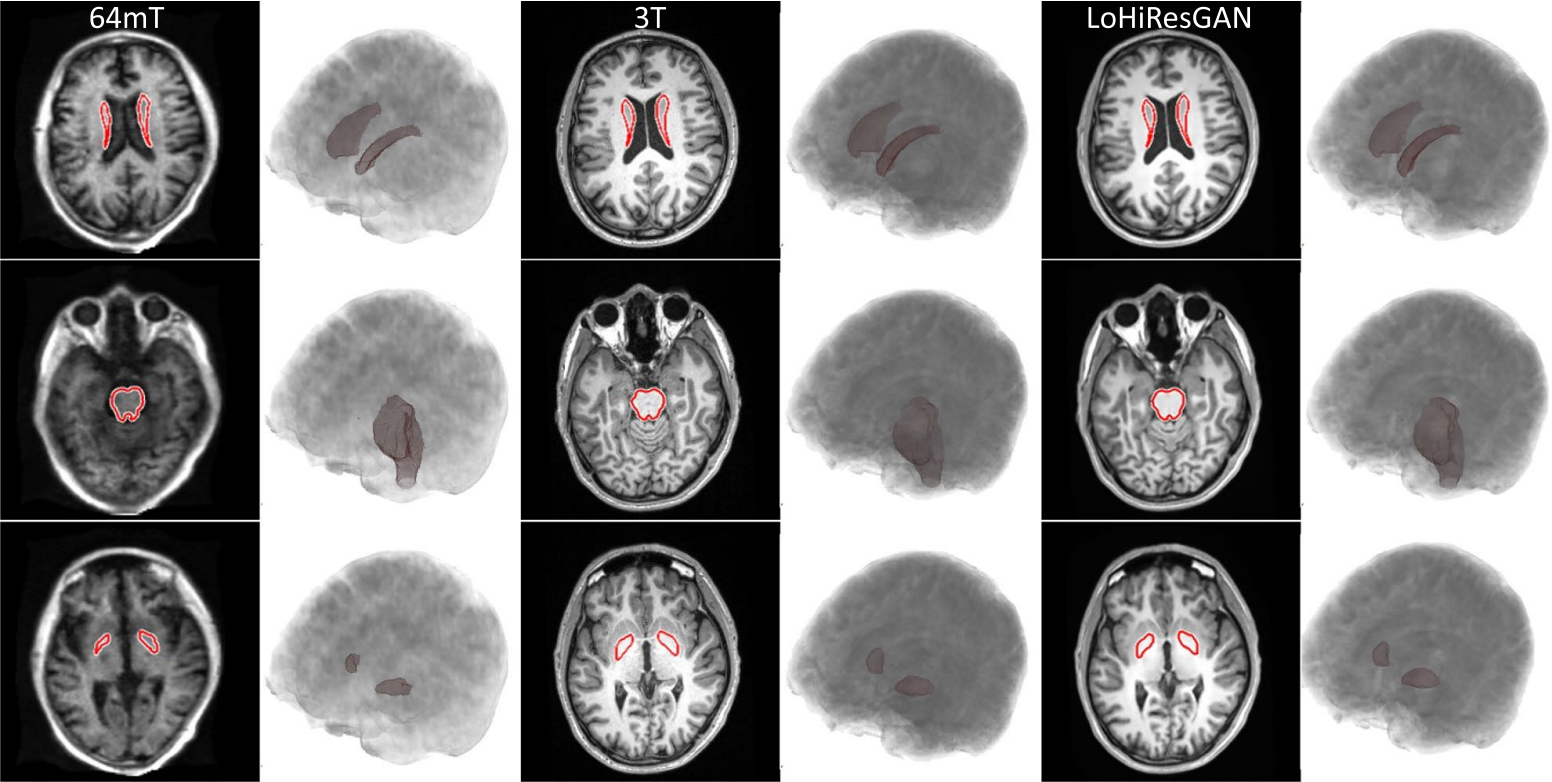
T1-weighted images showcasing the caudate nucleus, brain stem, and globus pallidus in rows one, two, and three, respectively, with their 3D renderings from a 26-year-old male.
